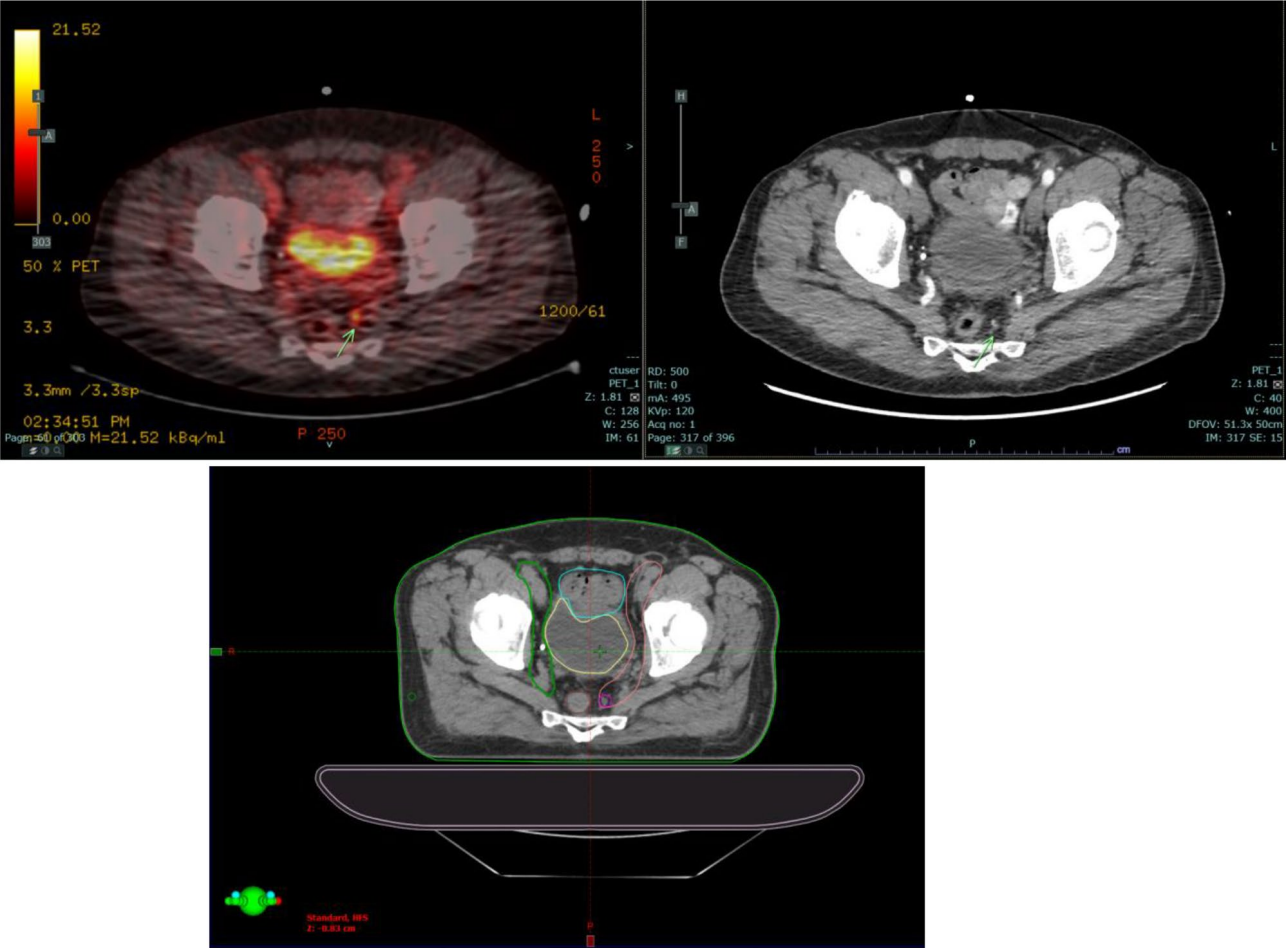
Example of PSMA PET positive mesorectal node not enlarged by CT criteria (3 mm) in which the radiotherapy contour was adjusted to cover the lymph node region when compared to the consensus atlas (Fig. 3, pictures G, H and I, Reference 7)
